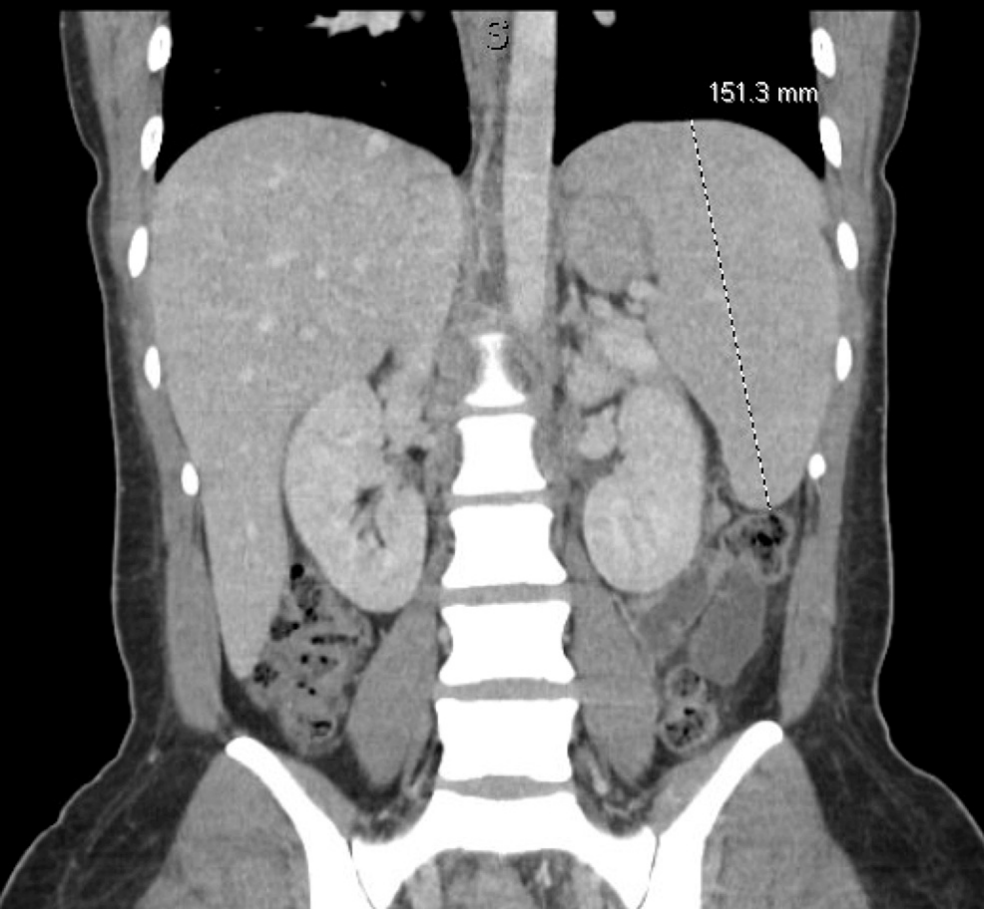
CT of the abdomen demonstrating splenomegaly.
